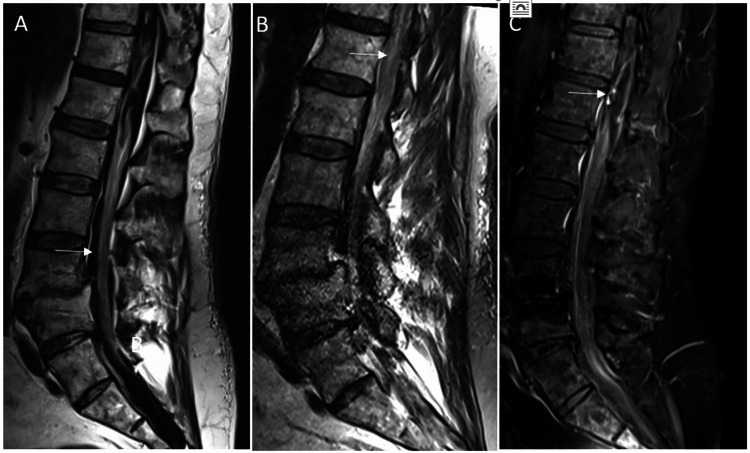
Sagittal MRI images from case three (A) T2 sequence showing the intradural hematoma (white arrow); (B) T2 sequence showing hyperintensity at the conus, consistent with a possible infarct (white arrow); (C) T1 post-contrast sequence showing abnormal enhancement in the ventral spinal canal (white arrow).
